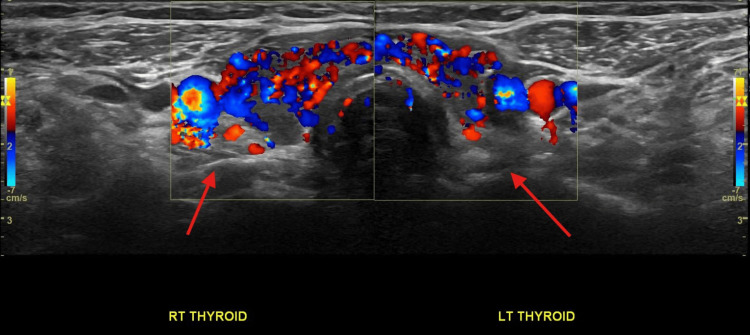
Thyroid ultrasound findings Color Doppler image demonstrating increased parenchymal vascularity, consistent with inflammatory thyroid disease.
